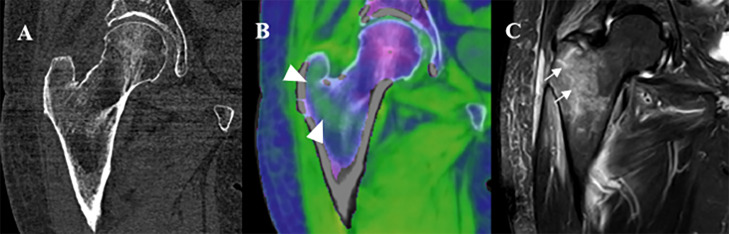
An 81-year-old woman consulted the emergency department with right hip pain after a low-energy trauma. (A) No clear fracture was visible on the grayscale images on the PCCT. (B) However, the bone marrow edema map shows intertrochanteric edema (white arrowheads), suggestive of a fracture. (C) MRI the following day confirms an intertrochanteric fracture (white arrows).
